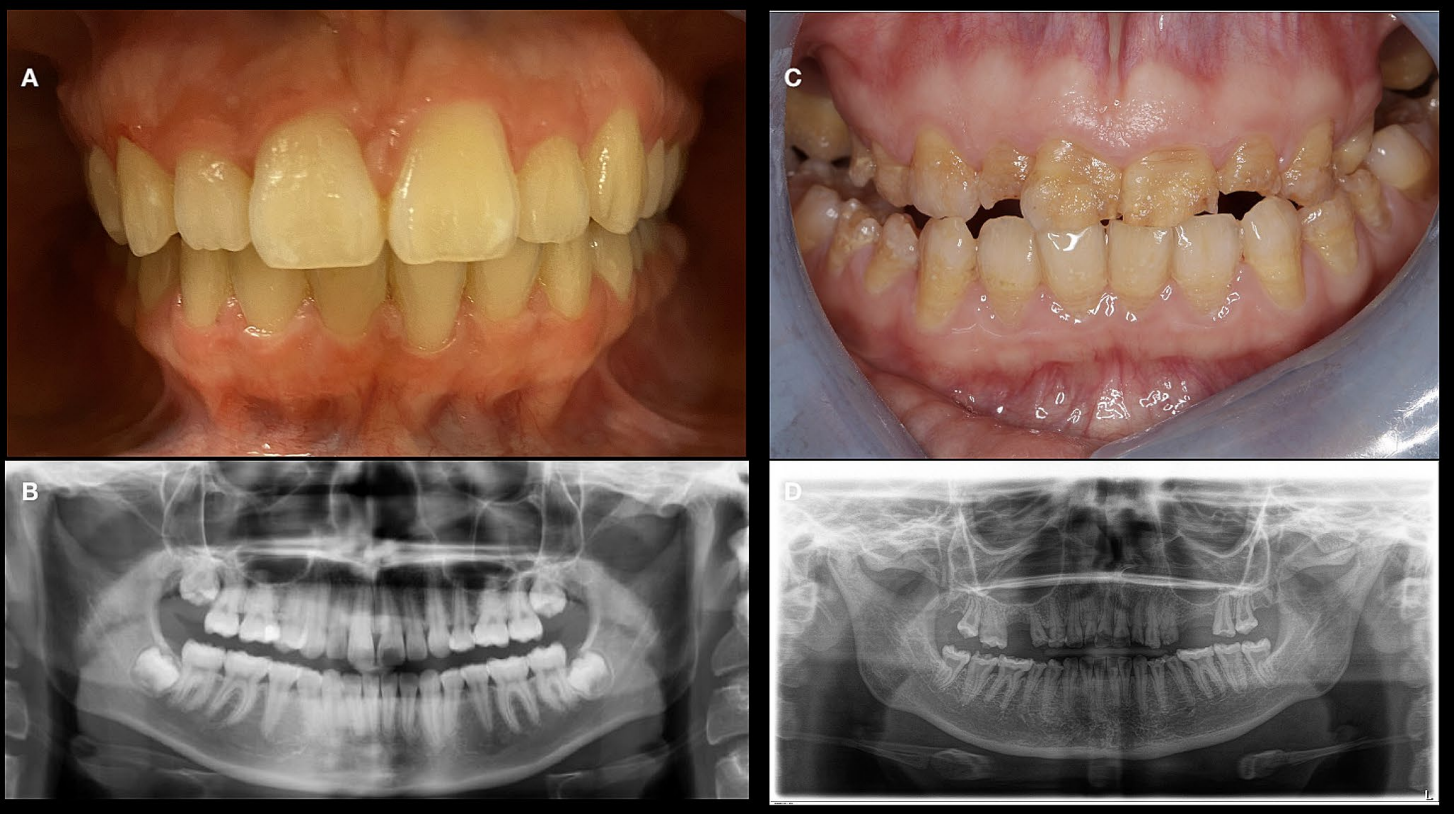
Clinical findings (Non-HPP patient vs. HPP patient). Direct comparison between Non-HPP patient (A, B) and HPP patient (C, D) based on clinical intraoral findings and associated panoramic radiograph
